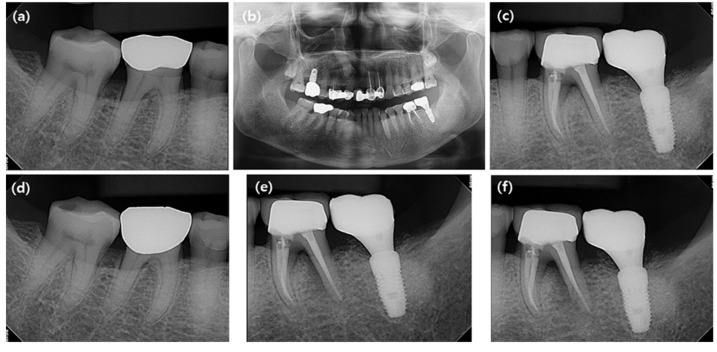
Radiographic measurement. (a) A periapical radiograph of the mandibular first molar (MnM1) adjacent the mandibular second molar (MnM2) at baseline. (b) A panoramic radiograph at baseline. (c) A periapical radiograph of MnM1 adjacent to the single implant placed in the mandibular second molar site (ImM2) at baseline. (d) A periapical radiograph of MnM1 adjacent MnM2 at the last follow-up. (e) A periapical radiograph of MnM1 adjacent to ImM2 at 3-year follow-up after prosthesis delivery of ImM2. (f) A periapical radiograph of MnM1 adjacent to ImM2 at the last follow-up.
